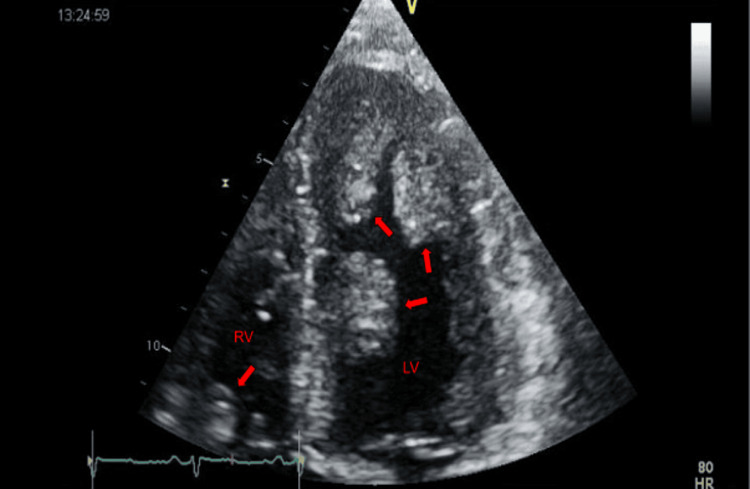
2D echocardiogram, apical four-chamber view with masses in the left ventricular (LV) and right ventricular (RV) cavities.
